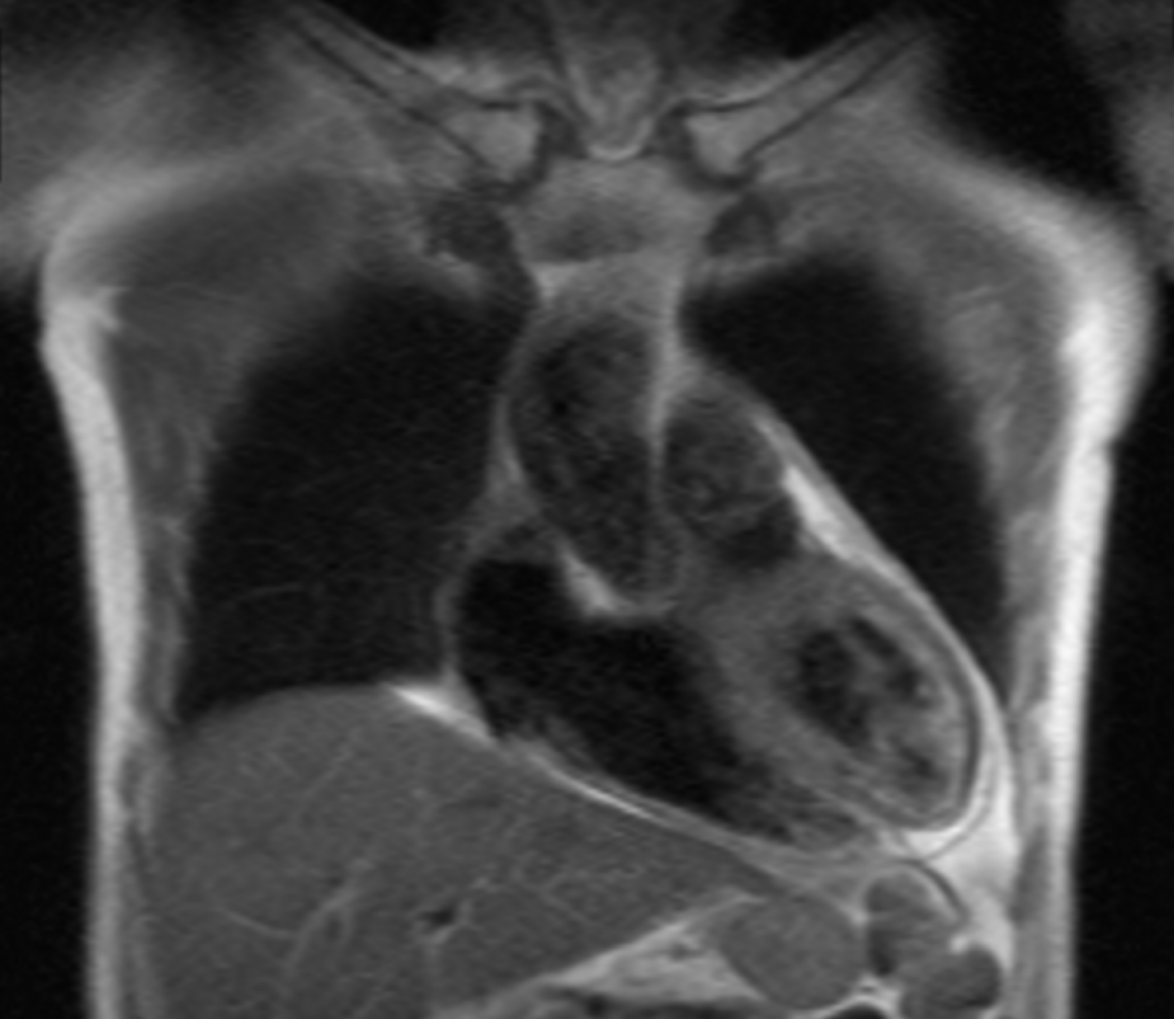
MRI of cardiac showing thickening of pericardium suggestive of constrictive pericarditis.
